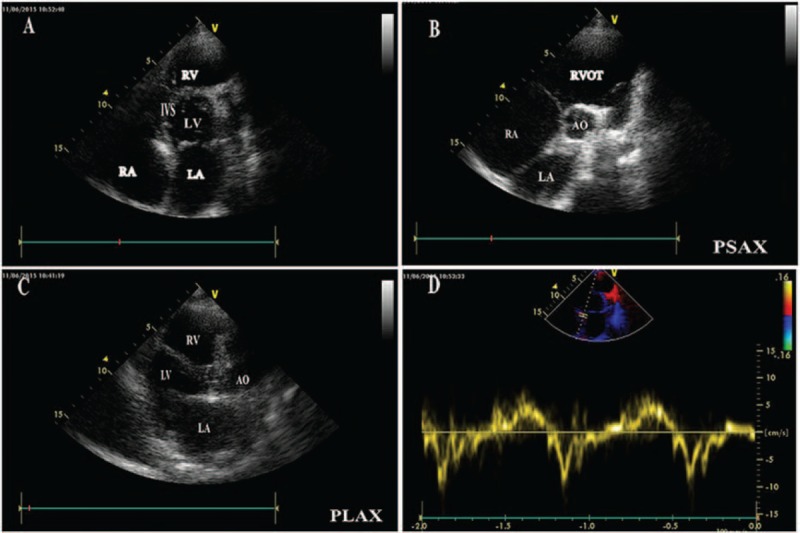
Transthoracic 2-dimensional echocardiogram images. (A) Four-chamber view shows the right ventricular dilation (right ventricle = 36 mm), biatrial enlargement, especially the massive right atria (left atrium = 48 mm, right atrium = 59 mm). In contrast, the left ventricle (LV) is smaller than right atria (LV = 44 mm). The whole view shows a special cardiac contour. (B and C) Both parasternal short-axis and long-axis views show right ventricular dilation associated with the markedly enlarged right ventricular outflow tract = 47 mm. (D) The tissue Doppler showed that the early diastolic septal mitral annular velocity was 10 cm/s.
